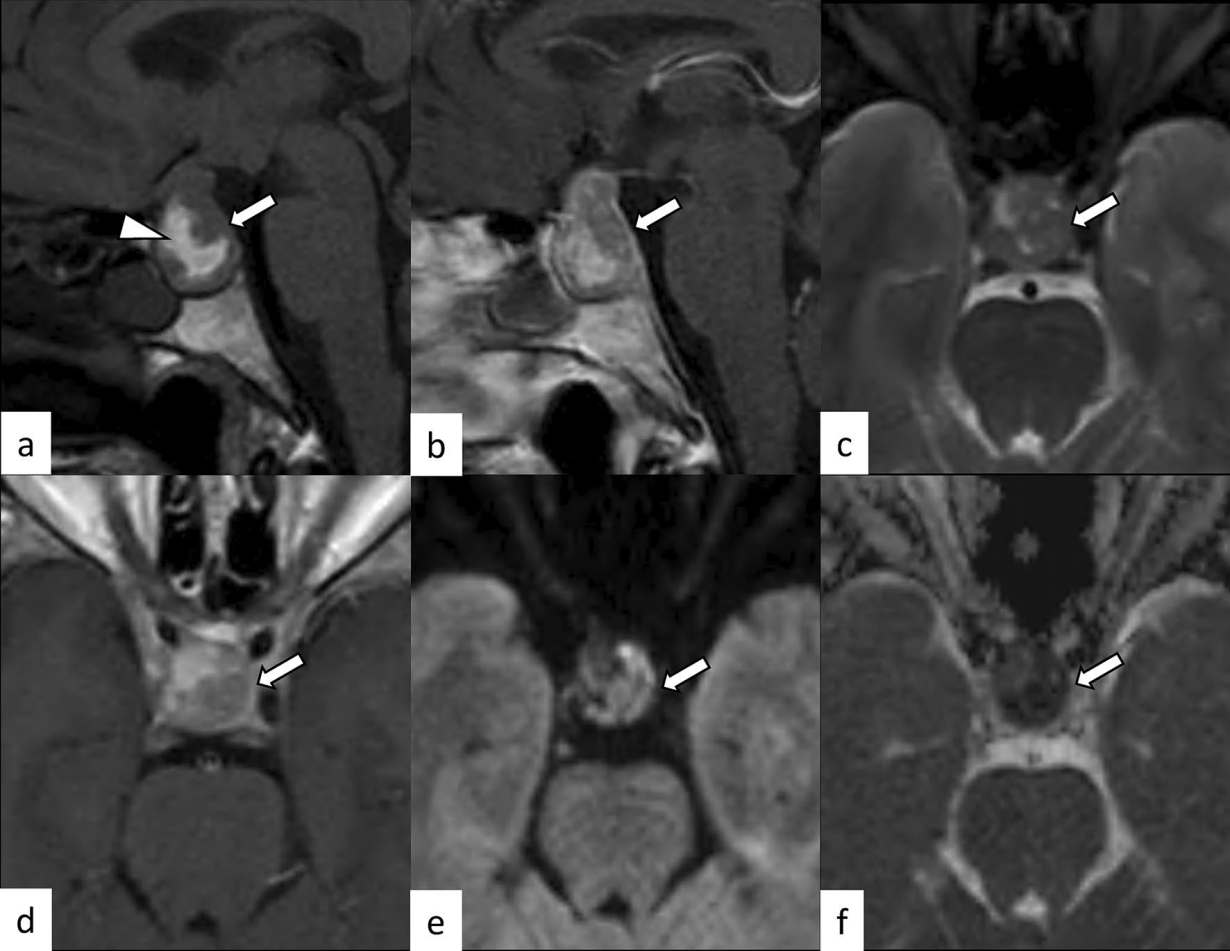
A 33-year-old woman with PitNET causing pituitary apoplexy. MRI shows a snowball-shaped mass extending from within the sella turcica to the suprasellar region (a–f, arrows). The mass has non-enhancing T1-weighted hyperintense components (a, arrowhead), suggestive of hemorrhagic changes, and enhancing peripheral components with T2-weighted isointensity c and restricted diffusion (e, f)
